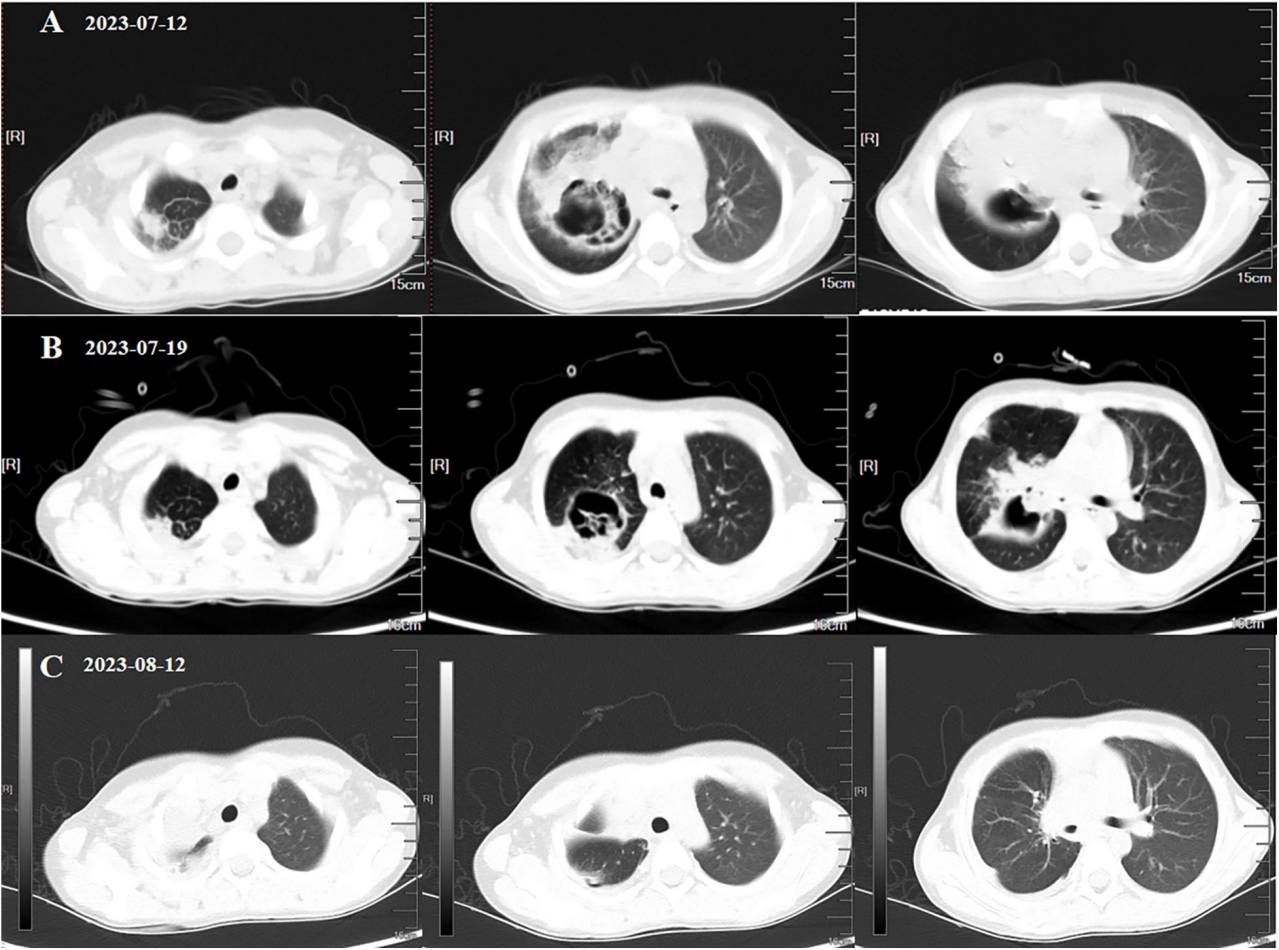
Chest CT imagings at different timelines of antibiotic therapy and surgery. (A) A chest CT scan taken before isavuconazole therapy. (B) A chest CT scan following 7 days of isavuconazole therapy. (C) A chest CT scan obtained 9 days postoperatively and following 28 days of isavuconazole therapy.
